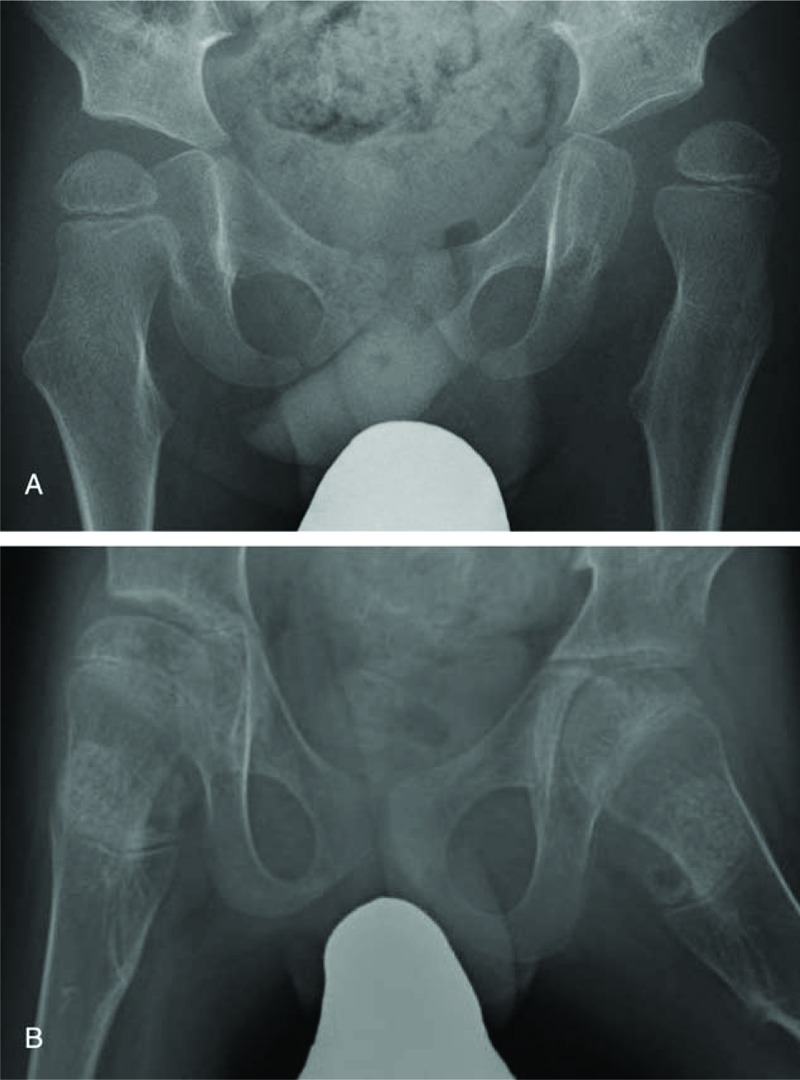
Radiographic representation of a patient with good prognosis before and after the soft tissue release surgery. Radiographs showing the hips of a boy with cerebral palsy, Gross Motor Function Classification System (GMFCS) level V. A, At 3 years of age with a migration percentage (MP) of 23% on the right side and 60% on the left side, acetabular index (AI) of 20.5° on the right side and 23.4° on the left side. B, 12 Years after soft tissue release, MP was 20% on the right side and 19% on the left side, with AI of 13.4° on the right side and 9.6° on the left side.
